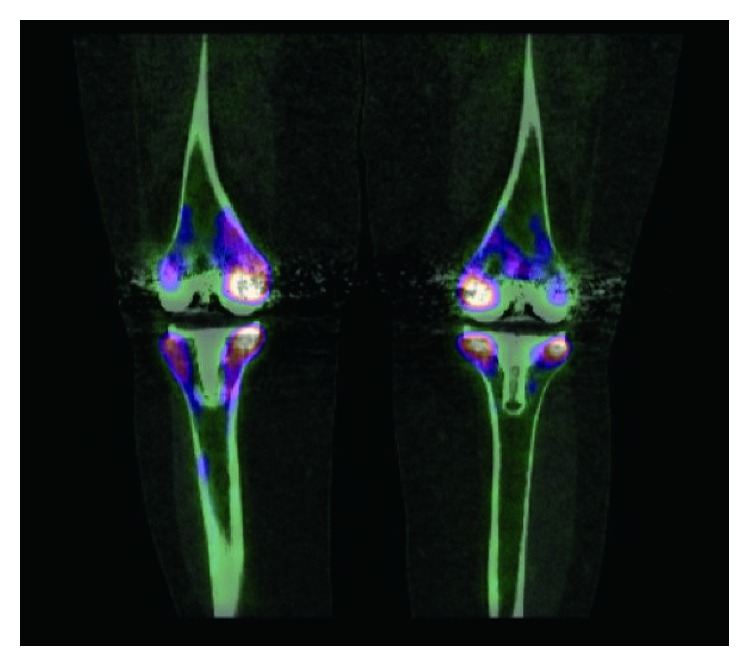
SPECT/CT image of bilateral prosthetic knees demonstrating increased radiotracer uptake around both knee joint prostheses compatible with periprosthetic joint infection.
